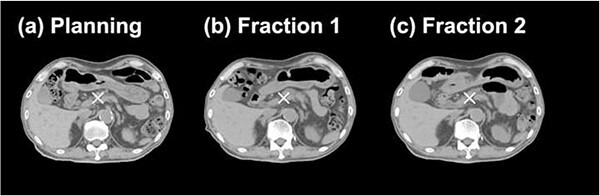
Axial slices including isocenter of (a) pCT images, and dCT images at (b) fraction 1 and (c) fraction 2 for Patient 2. The dCT images were aligned by the fiducial marker position. The white crosses indicate the isocenter on each CT image.
